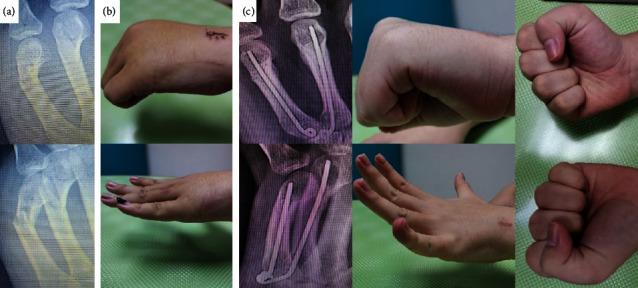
A 19-year-old male, with fracture of the fourth and fifth metacarpal shafts: (a) preoperative radiographs, (b) 2 weeks postoperative range of motion, (c) at 6 weeks after the operation, the patient's radiographs showed a sign of bone union. He regained excellent ASSH TAF score. His TAF of his left ring finger was 265 degrees (normal: 270). His grip strength was 71.15% of the contralateral hand. His DASH score was 14.16. He had lost to follow-up after implant removal.
